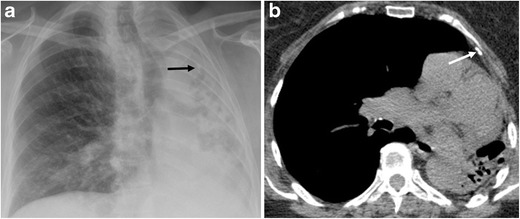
Same patient as in Fig. 4. (a) Chest radiograph shows total unilateral left lung destruction and calcification projected on the upper half of left hemithorax. (b) Non-enhanced CT confirms the presence of left upper pleural calcification in correspondence with the calcification seen on chest radiograph
